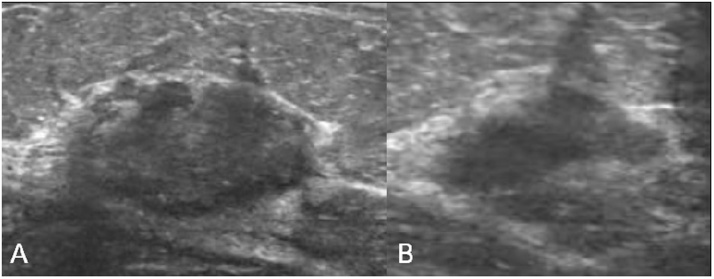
Ultrasound transverse (A) and sagittal (B) scans showing an hypoechoic inhomogeneous nodular lesion corresponding to the breast lump on the surgical scar at the subareolar region of the left breast.
